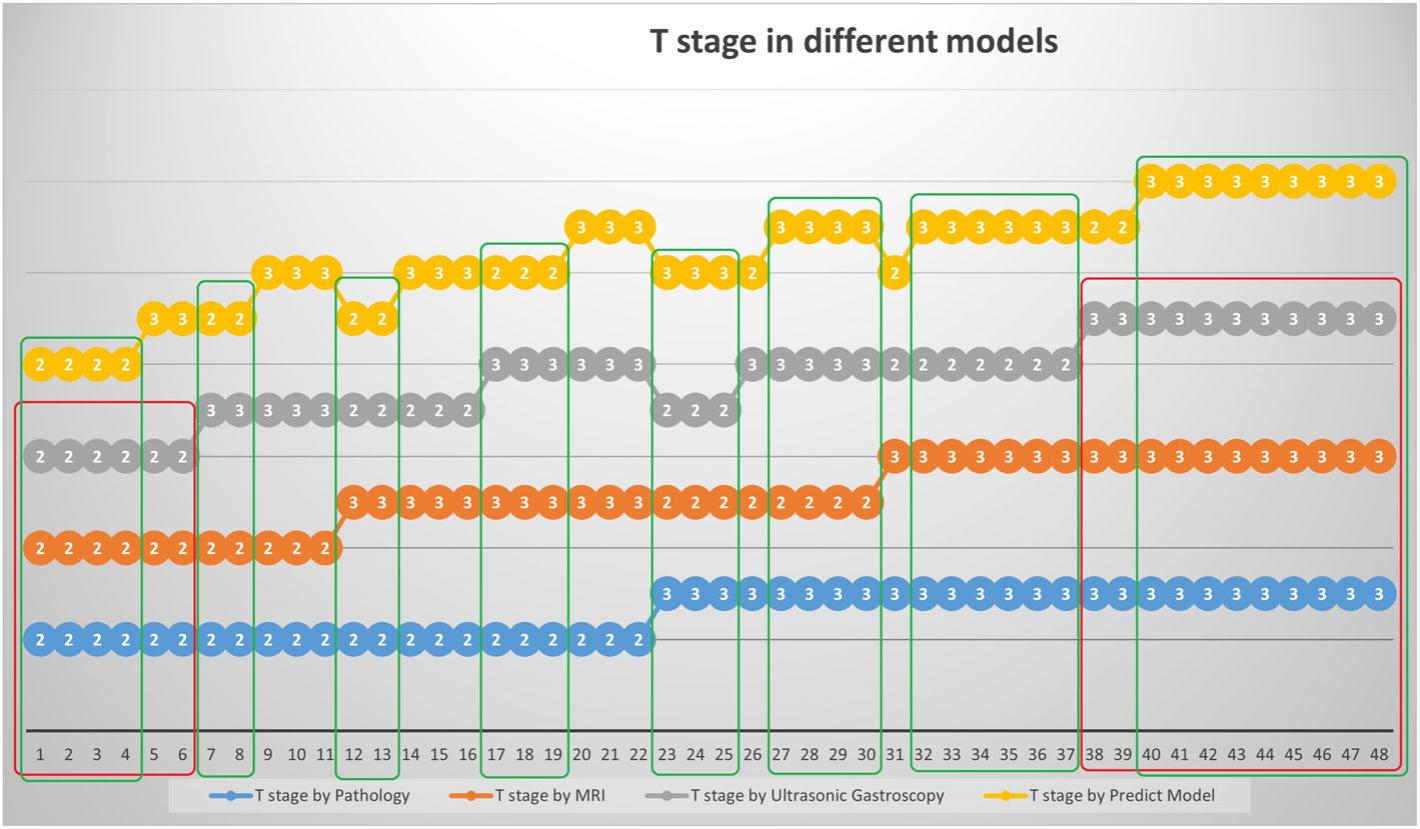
One‐to‐one correspondence of the T stage prediction methods for each patient under different staging modes. The patients in the green circle are those who were accurately judged by the prediction model, and the patients in the red circle are those whose ultrasound T stage and magnetic resonance imaging (MRI) T stage are the same and accurately judged.
